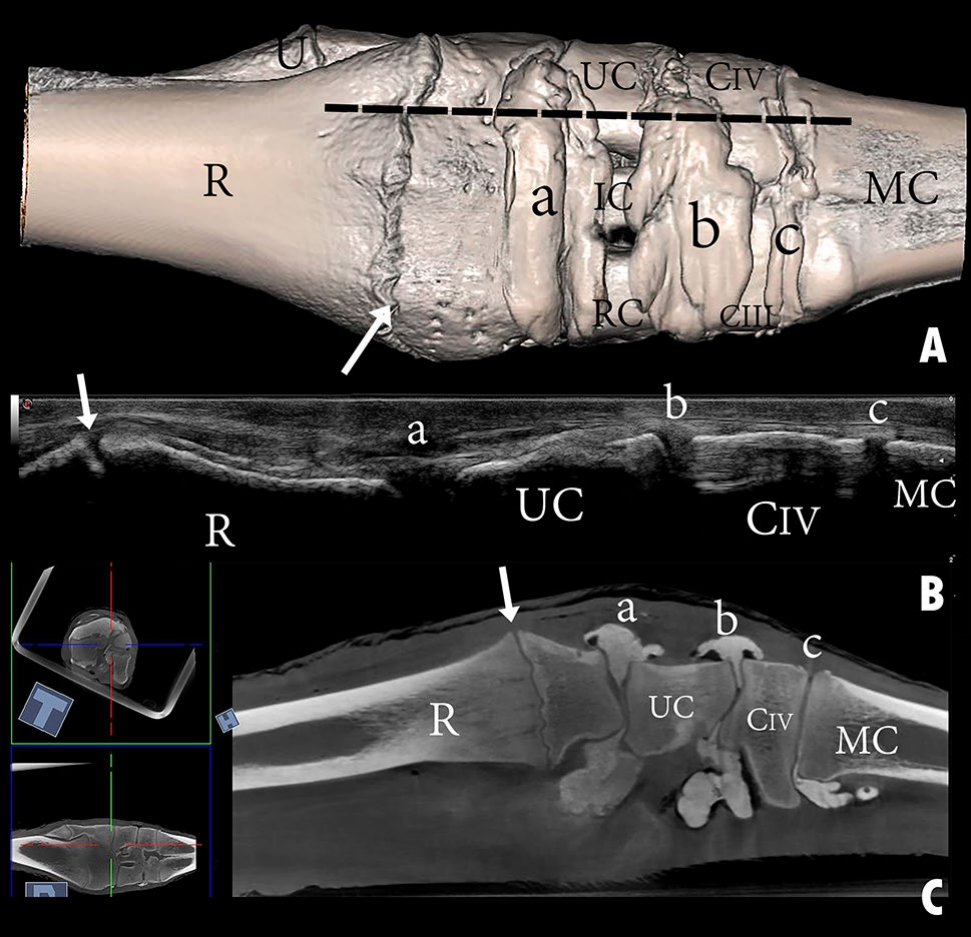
Dorsal view of a left carpal joint showing (A) three-dimensional reconstruction of the carpal joint (the black dotted line indicates the dorsolateral placement of the ultrasound probe); (B) longitudinal composed ultrasound image of the carpal joint; (C) sagittal plane computerized tomography image. R, the distal end of the radius; U, the distal end of the ulna; RC, radiocarpal bone; IC, intermediate carpal bone; UC, ulna carpal bone; CIII, carpal bone III; CIV, carpal bone IV; MC, metacarpal bone; a, radiocarpal joint cavity; b, intercarpal joint cavity; c, carpometacarpal joint cavity. The white arrow indicates the epiphysial line.
